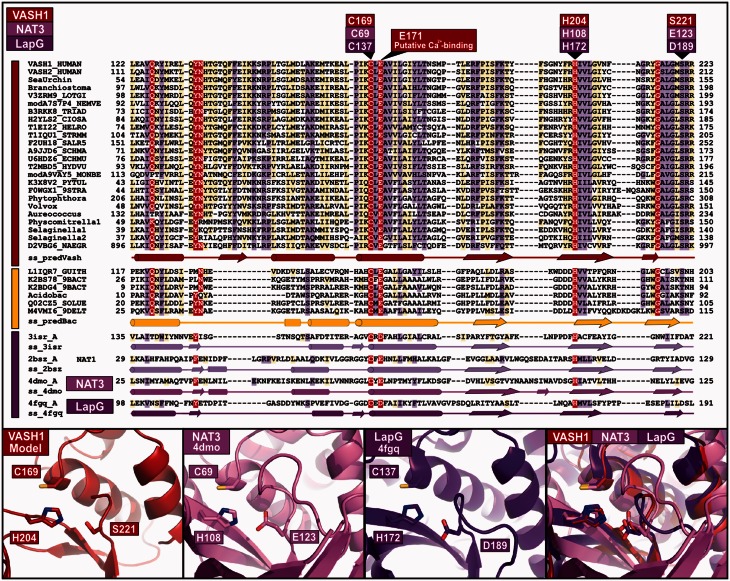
Multiple sequence alignment of representative vasohibin and related families. Multiple sequence alignments for each family were generated with the program T-Coffee (Notredame *et al*., 2000) using default parameters and slightly refined manually. The final superfamily alignment was generated using a combination of profile-to-profile comparisons (Söding *et al*., 2005) and sequence alignments derived from structural super-positions for those families whose tertiary structure is known (PDB IDs: 3isr, 2bsz, 4dmo and 4fgq) (Holm and Sander, 1995). Active site residues are labelled and coloured according to reference protein sequences: VASH1, NAT3 (human arylamine N-acetyltransferase 3), and calcium-dependent periplasmic cysteine protease LapG in red, pink and violet, respectively. A putative calcium-binding residue (human VASH1 E171) is labelled. Families are indicated by coloured bars to the left of the alignment: Vasohibin, Vasohibin-like and transglutaminase-like cysteine protease are indicated in red, yellow and purple, respectively. The limits of the protein sequences included in the alignment are indicated by flanking residue positions. Secondary structure predictions (Jones, 1999) were performed independently for the vasohibin and vasohibin-like families (show in ss_predVash and ss_predBac lanes); these are consistent with X-ray determined secondary structures of the putative cysteine protease from *C. hutchinsonii* (PDB: 3isr) (Stein *et al*., *Midwest Center for Structural Genomics*, unpublished), NAT1 (arylamine N-acetyltransferase-1) from *Mesorhizobium loti* (PDB: 2bsz) (Holton *et al*., 2005), NAT3 (arylamine N-acetyltransferase-3) from *Bacillus cereus* (PDB: 4dmo) (Kubiak *et al*., 2013) and the calcium-dependent periplasmic cysteine protease LapG from *Legionella pneumophila* (PDB: 4fgq) (Chatterjee *et al*., 2012). Alpha-helices and beta-strands are indicated by cylinders and arrows, respectively. The alignment was presented with the program Belvu using a colouring scheme indicating the average BLOSUM62 scores (which are correlated with amino acid conservation) of each alignment column: red (>3), violet (between 3 and 1.5) and light yellow (between 1.5 and 0.5) (Sonnhammer and Hollich, 2005). Sequences are named according to their UniProt identification or common name (details provided in Supplementary Figure S1). Below the alignment are shown a homology-model of human VASH1 and known structures of representative members of the transglutaminase-like cysteine protease superfamily. Catalytic triads are labelled and side chains shown using sticks. The human VASH1 structural model was created using Modeller (Sali and Blundell, 1993). VASH1 model and structures are presented using Pymol (http://www.pymol.org)
